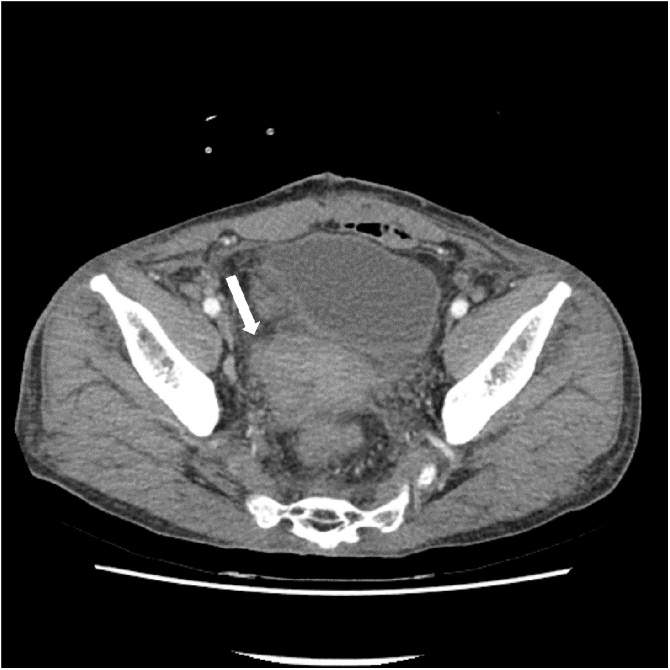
Follow-up contrast-enhanced axial computed tomography image showing the massive hematoma in the perirectal space, without any definite signs of extravasation.
